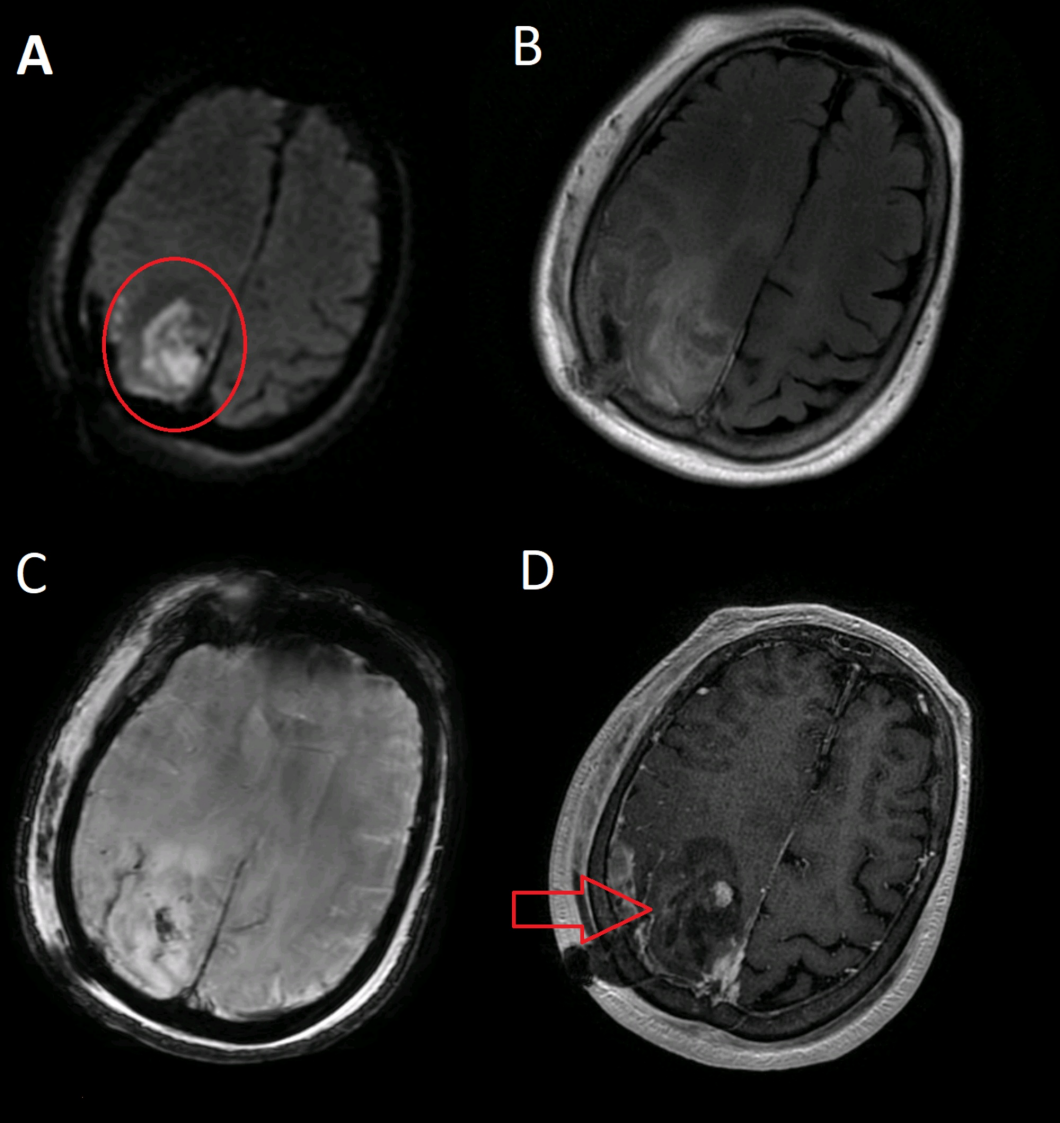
MRI of the brain with and without contrast (A-D) Right parieto-occipital glioma with defined margins (red circle and arrow)
